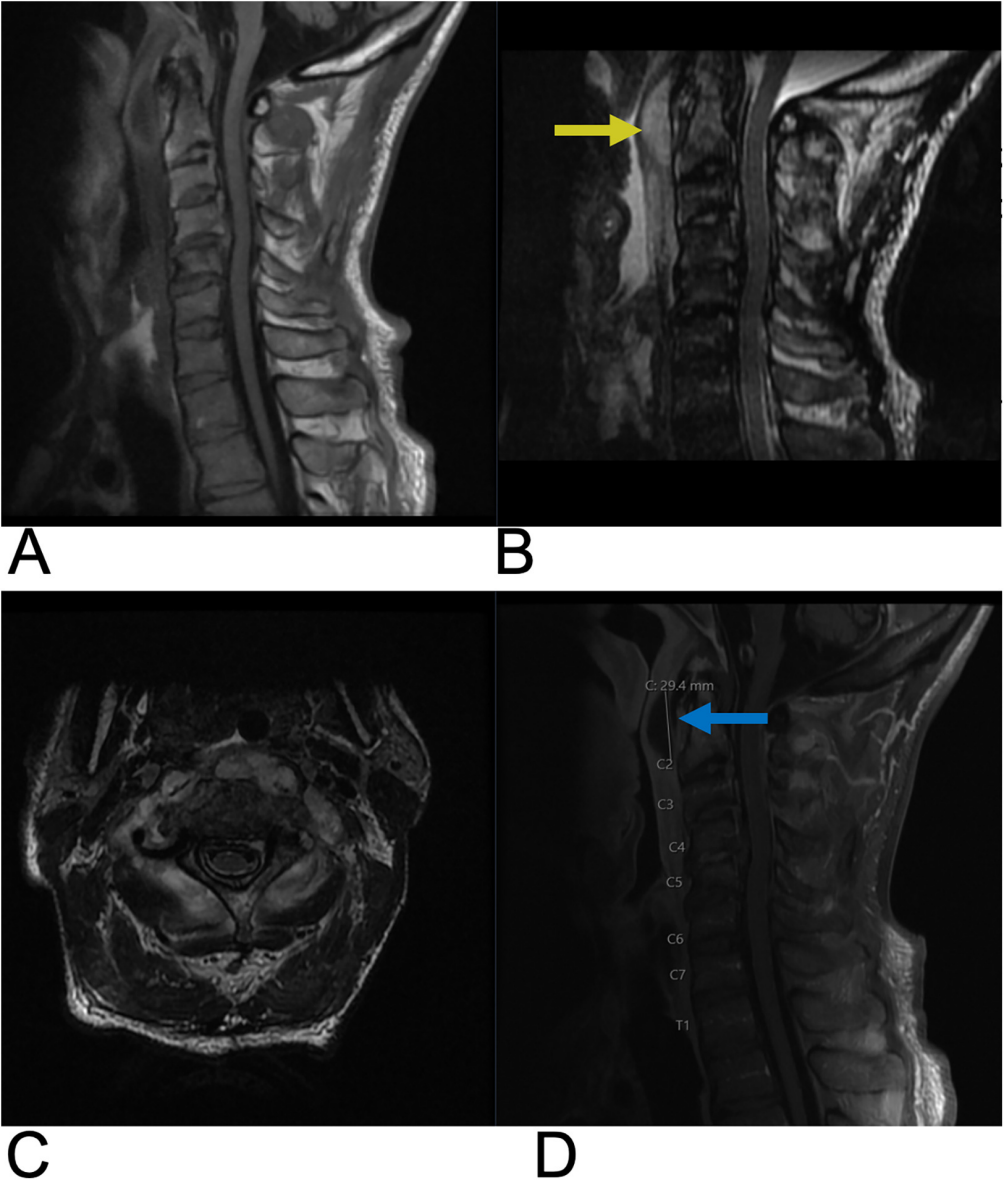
Retropharyngeal abscess. Sagittal T1 (A), sagittal T2 (B), axial T1 (C), and sagittal T1 + C (D) MRI of the cervical spine demonstrating a retropharyngeal abscess appearing as a T2 hyperintense fluid collection (yellow arrow), with peripheral enhancement (blue arrow) located posterior to the pharynx and anterior to the cervical spine with significant prevertebral soft tissue swelling causing displacement of the spinal cord at the C3–C4 level.
